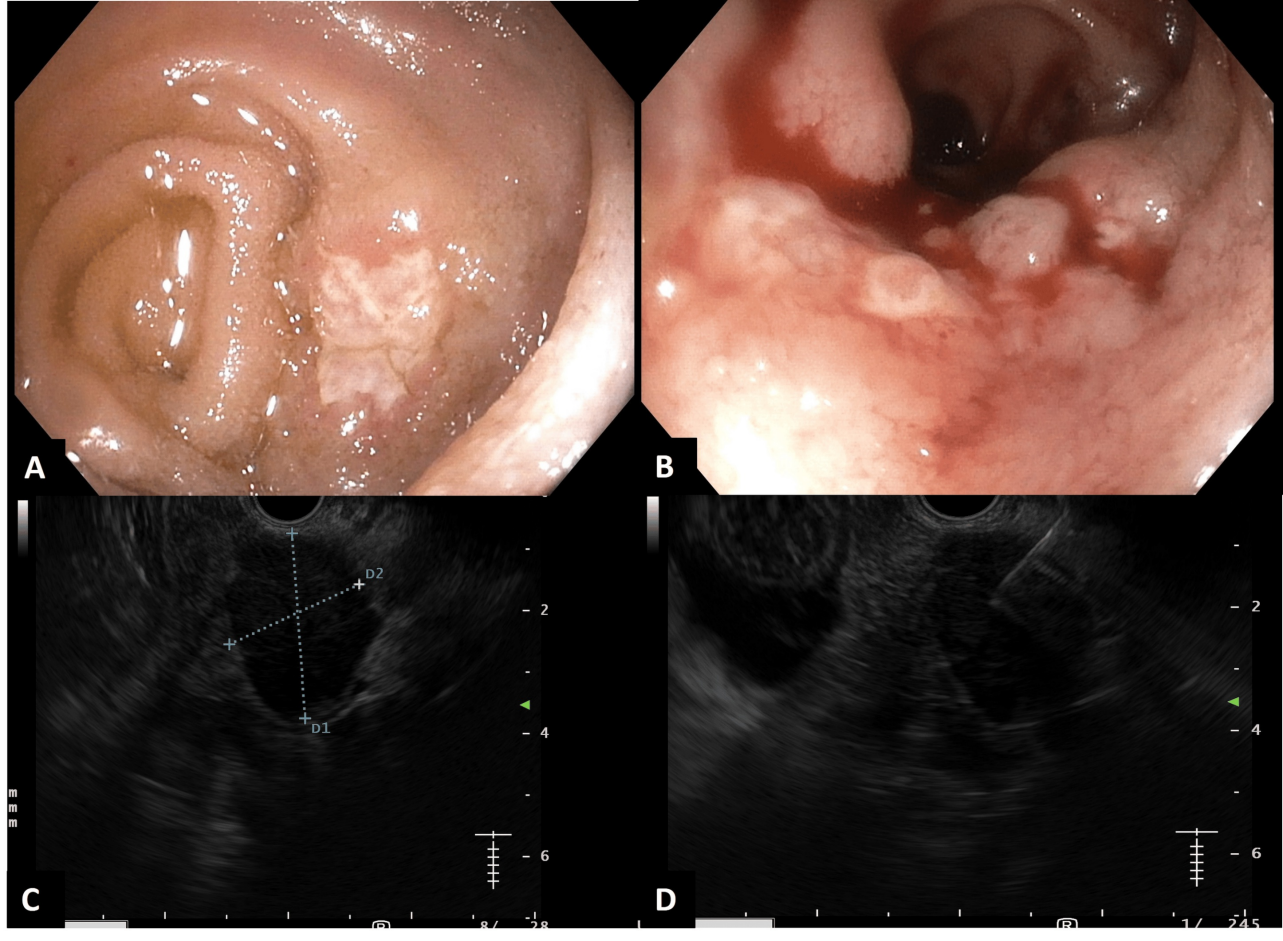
Colonoscopy image shows (A) superficial, round ulcers in the terminal ileum. (B) Nodular mucosal changes are also seen in the terminal ileum. (C) Endoscopic ultrasound (EUS) reveals a large, hypoechoic, oval lymph node in the peripancreatic region. (D) Fine-needle biopsy is obtained under EUS guidance.
